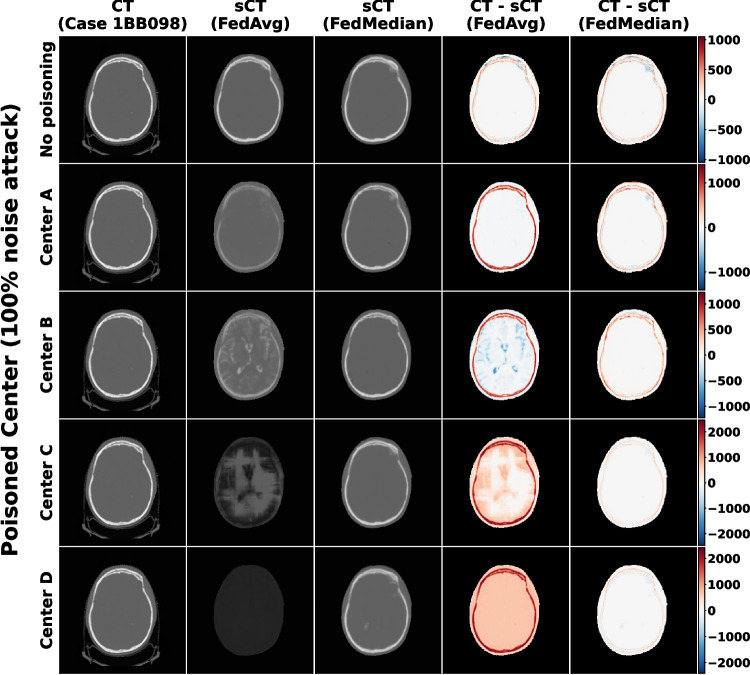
Visual comparison of sCT for a representative patient from external Center E under a 100% noise data poisoning attack ($$\gamma = 1.0$$), corresponding to the worst-case scenario. The first row represents the no-poisoning baseline, while the other rows correspond to a different poisoned training center (Centers A-D). Columns show, from left to right, the reference CT, the sCT generated with FedAvg and FedMedian aggregation, and the corresponding pixel-wise difference maps (CT − sCT). FedMedian consistently restored image quality across all poisoning configurations, while FedAvg produced severely corrupted and invalid outputs
